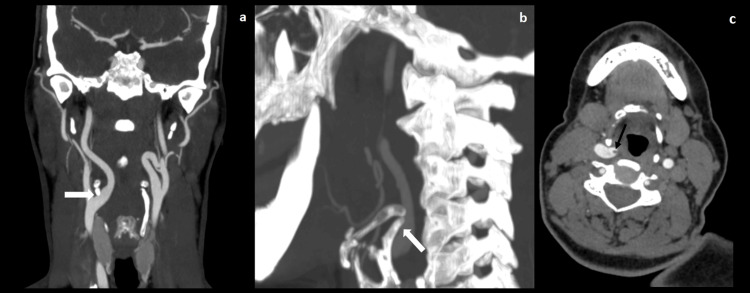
Case 4 frontal (a), sagittal (b), and axial (c) CT scans demonstrating compression of the right ICA by the greater horn of the hyoid bone (white arrows) complicated by a floating thrombus (black arrow) CT: computed tomography, ICA: internal carotid artery
